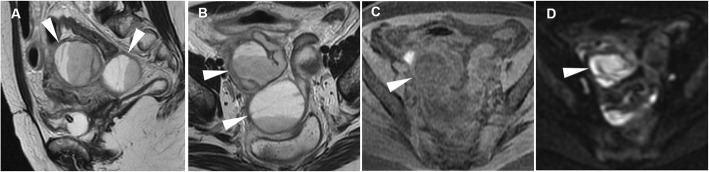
Acute pyosalpinx in a 44-year-old woman with a history of surgically treated pelvic endometriosis 5 years earlier, suffering from abdominal pain, fever and diarrhoea. Sagittal (a), axial (b) T2-weighted images and axial fat-suppressed T1-weighted (c) image show a distended right fallopian tube (arrowheads) with thickened walls and internal fluid-fluid levels. On axial DWI (*b* = 800 s/mm^2^) image (d), the involved fallopian tube (arrowhead) demonstrates high signal intensity reflecting restricted diffusion from purulent content
